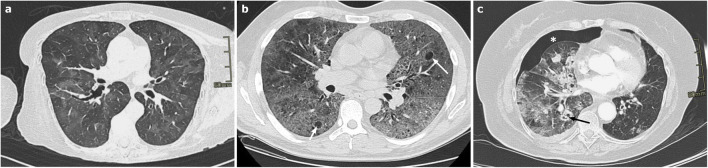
a–c Axial HRCT images of three patients with different stages of Pneumocystis jiroveci pneumonia showing, at an early stage (a), bilateral, diffuse ground-glass opacities with a mosaic attenuation pattern; and in advanced stages (b–c) more extensive ground-glass opacities and parenchymal, variable in shape and size cysts (arrows in b and c), whose rupture can sometimes lead to pneumothorax (white * in c)
